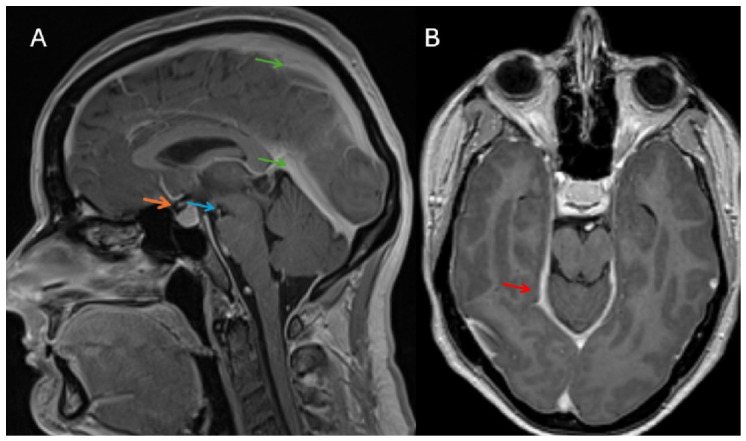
Post-contrast T1-weighted MRI images in sagittal (A) and axial (B) planes obtained after intravenous gadolinium administration. The images demonstrate multiple signs of intracranial hypotension. The red arrow indicates diffuse, smooth pachymeningeal enhancement. The orange arrow shows pituitary enlargement due to venous engorgement. The blue arrow marks reduced size of the suprasellar and prepontine cisterns. The green arrow highlights engorged venous sinuses. Image (B) shows a decreased ventricular size (not indicated by an arrow).
